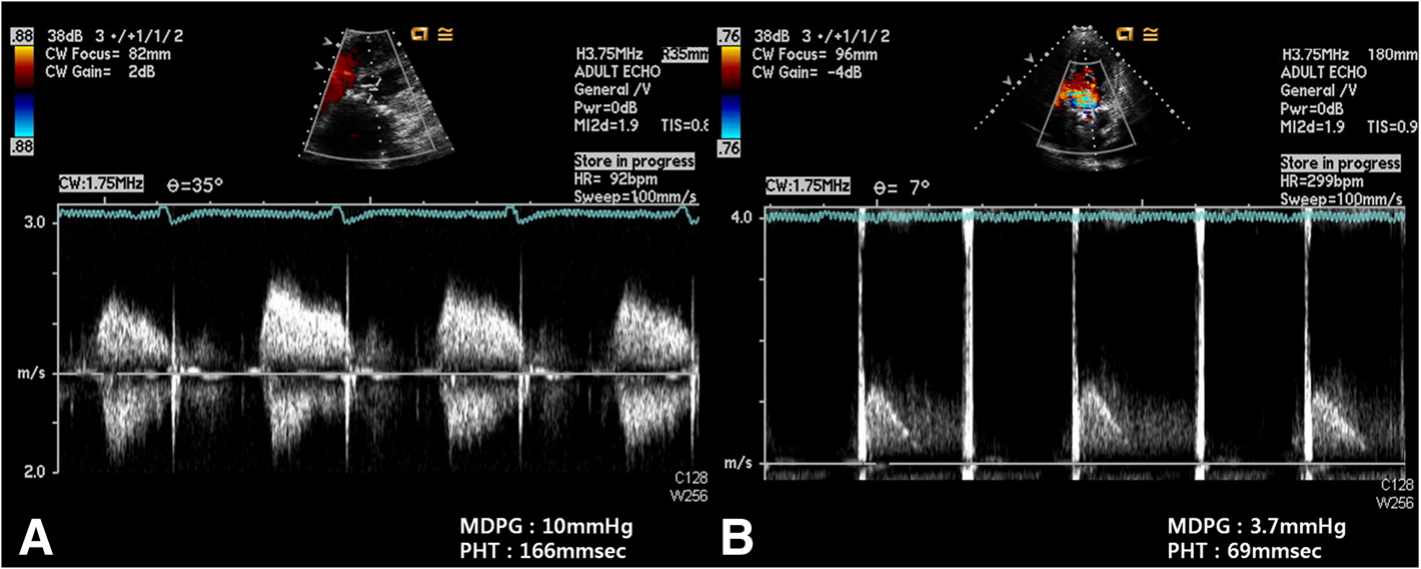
Transthoracic echocardiogram. An increased mean diastolic pressure gradient of 10 mmHg after BVR (A). Following reoperation, a comparable transthoracic image depicting normalization of mean gradient (B).
